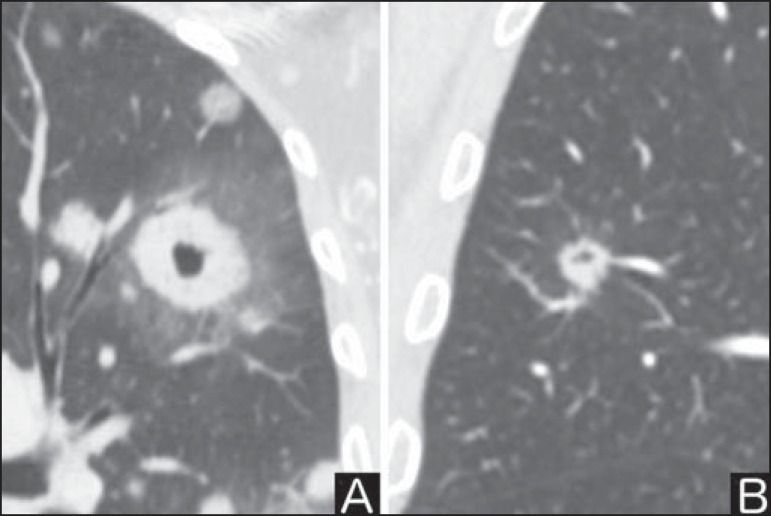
Excavation.* Chest CT - lung window. A:
